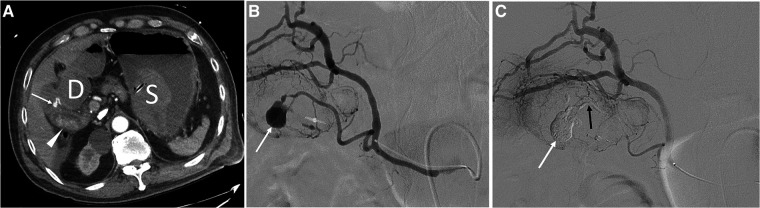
2 A 75-year-old male with hematochezia. (A) CT scan shows a pseudoaneurysm (arrow) located within the gallbladder. Note the presence of a blood clot with high attenuation in the stomach (S), duodenum (D), and gallbladder (arrowhead). (B) Right hepatic angiogram shows a pseudoaneurysm (arrow) from the superficial cystic artery. A mixture of n-butyl cyanoacrylate and Lipiodol was used for embolization of the bleeding point. (C) The final angiogram after embolization of the cystic artery shows a subtraction artifact of the pseudoaneurysm (white arrow) and superficial cystic artery (black arrow). Note intact perfusion of the deep cystic artery. The impacted gall stone was thought to induce development of a fistula between the gallbladder and duodenum, resulting in duodenal ulcer and hematochezia. The patient recovered without development of ischemic cholecystitis and was discharged two weeks after cystic artery embolization.
